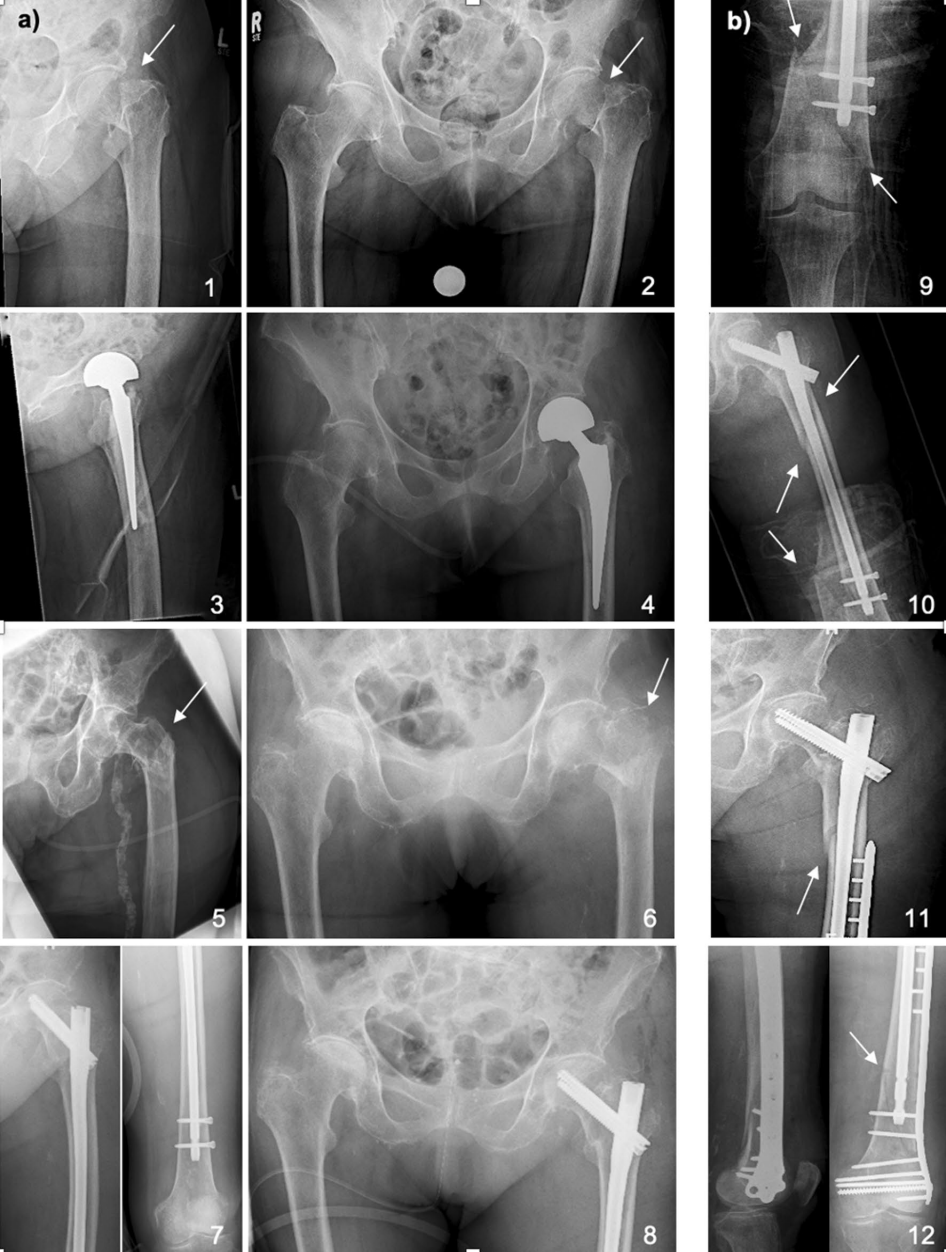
Operative intervention in patients presenting with (a) PFFs or (b) periprosthetic femur fracture. (a) First case^1–4^: A 94-year-old women was admitted to the ER with clinical signs of a PFF after falling at her private apartment. Radiographs of the left hip joint axial^1^ and the pelvis^2^ showed a medial femoral neck fracture (arrows). Due to already impaired walking ability and osteoporosis, a cemented hemi-arthroplasty was implanted. The patient recovered for 4 days at our intensive care unit (ICU). Radiographs 3 and 4 show postoperative results on day two. The patient was able to return home after specialized geriatric rehabilitation. Second case^5–8^: A 97-year-old women with dementia was administered to our ER after falling during transition at her assisted living facility. Radiographs of the left hip joint axial^5^ and the pelvis^6^ showed a multifragmentary pertrochanteric fracture (arrows). After closed reduction and internal fixation using a femur nail, the patient spent 24 h at the ICU. Postoperative radiographs 7 and 8 show results on day two. The patient was discharged into specialized geriatric rehabilitation. (b) At the rehabilitation facility the patient from case 2 refused weight bearing after a few days with initial walking ability and an unobserved fall was suspected. Radiographs of the pelvis and femur showed a distal supracondylar multifragmentary periprosthetic femur fracture with a rotary wedge reaching the subtrochanteric region (see arrows in image 9 and 10). Osteosynthesis to stabilize the distal fracture fragment was performed using a plate and postoperative recovery took place for two days at our ICU. Images 11 and 12 show postoperative results on day two. Due to prolonged recovery and increased need for care the patient was discharged to a short-term-care facility needing higher nursing intensity.
